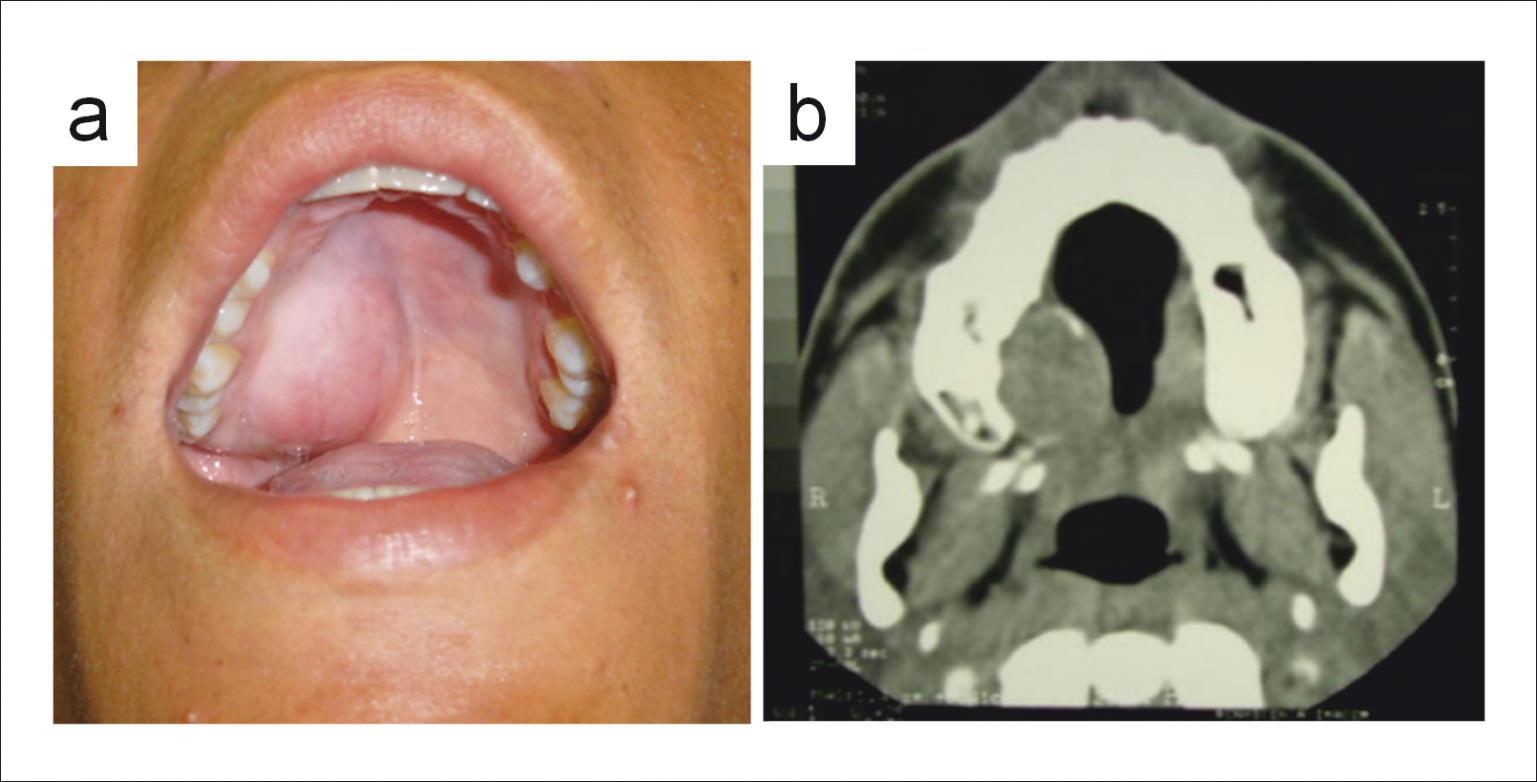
Clinical and imaging features. (a) Intraoral swelling in the right side of the hard palate. (b) Computed tomography showing large hypodense tumor provoking slight erosion of maxillary cortical bone.
